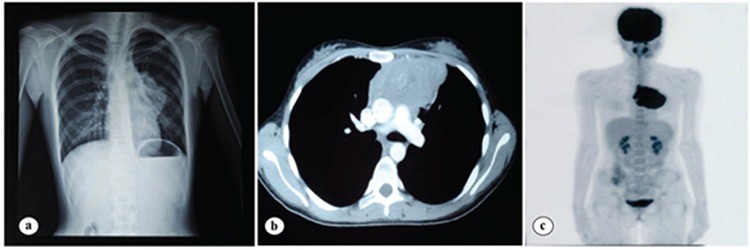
Radiological findings of primary mediastinal B-cell lymphoma. a) Appearance of the left parahilar mass on chest plain film. b) Thorax computed tomography depicts a mass of 10x9x5 cm in the anterior mediastinum with irregular lobulated borders invading the pericardium. c) Positron-emission tomography scan shows increased fludeoxyglucose uptake in the tumor.
